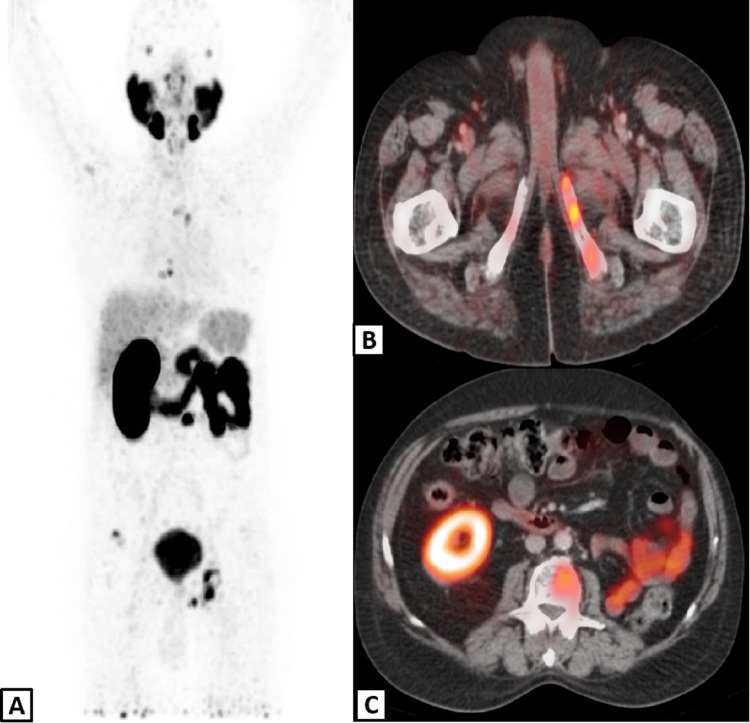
PSMA PET-CT scan Maximum intensity projection (A) and fused axial PET-CT slice (B) demonstrate multiple osseous metastases. The left kidney is not visualized in fused axial PET-CT slice at kidney level (C) due to nephrectomy.
